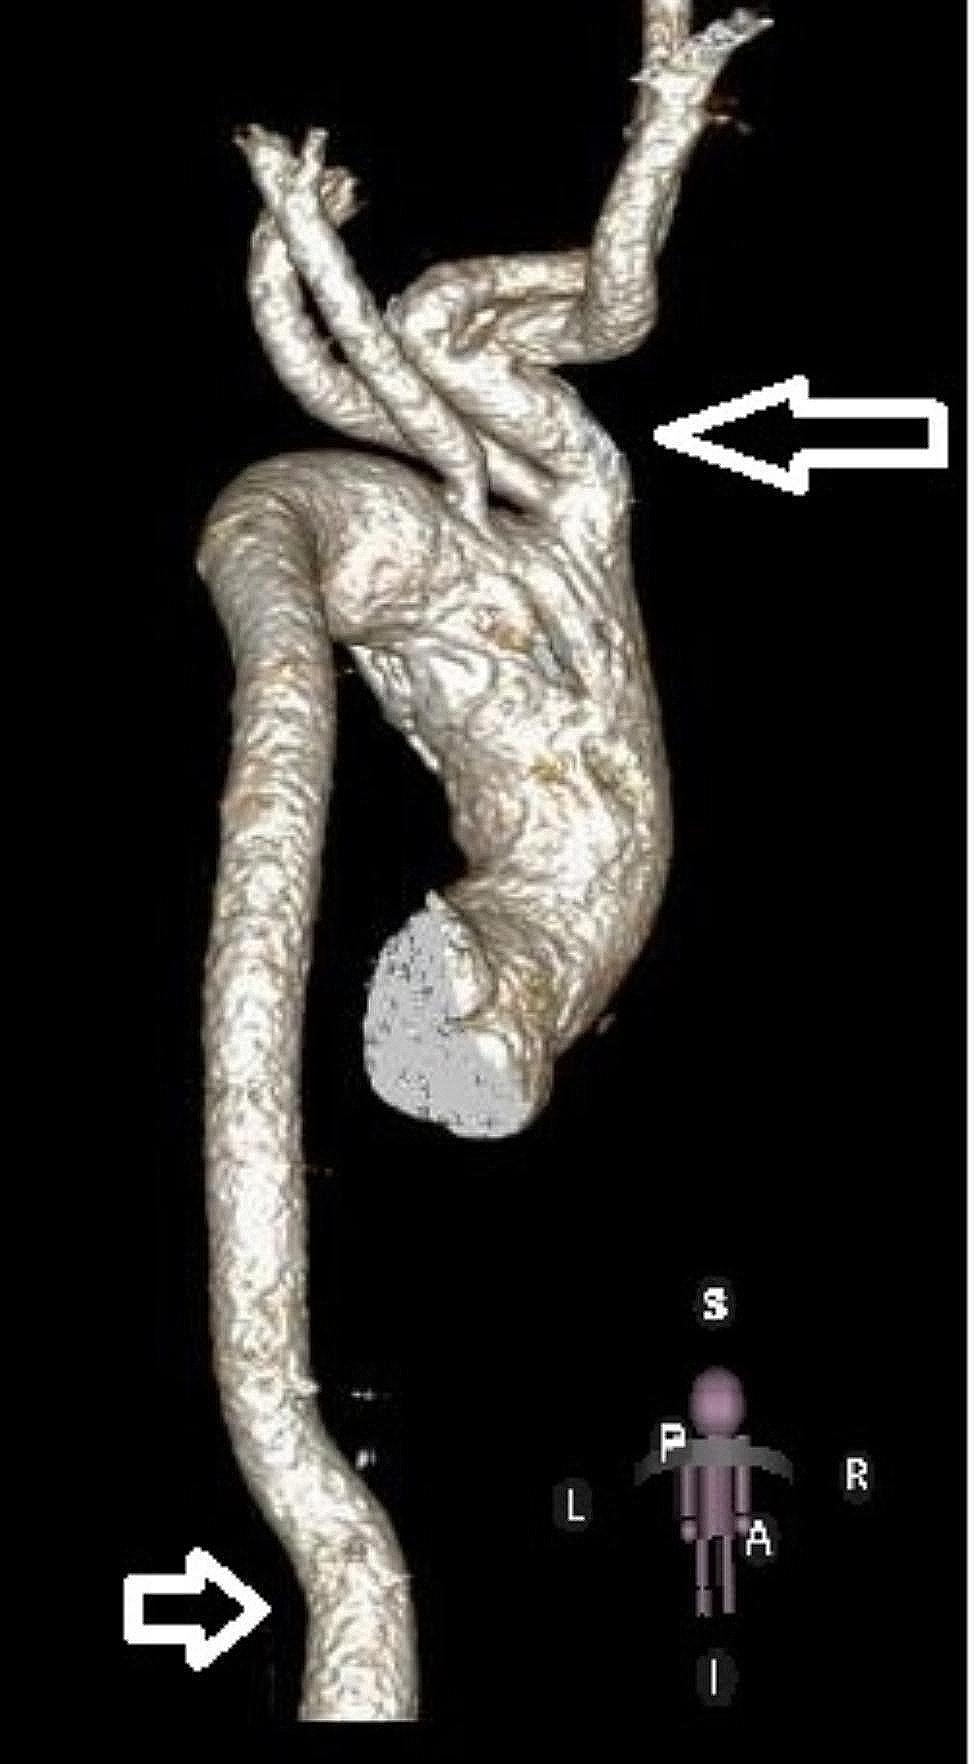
The 3-D reconstructed CT image shows a posterior view of the tortuous great vessels and the aorta (arrows). (A-anterior, P-posterior, S-superior, I-inferior, R-right, L-left)
